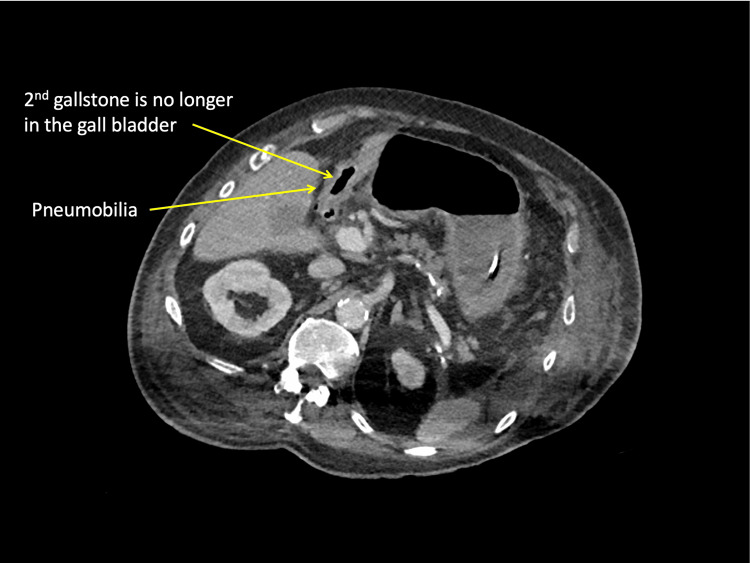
Axial CT showing inflamed thickened gall bladder containing air.
